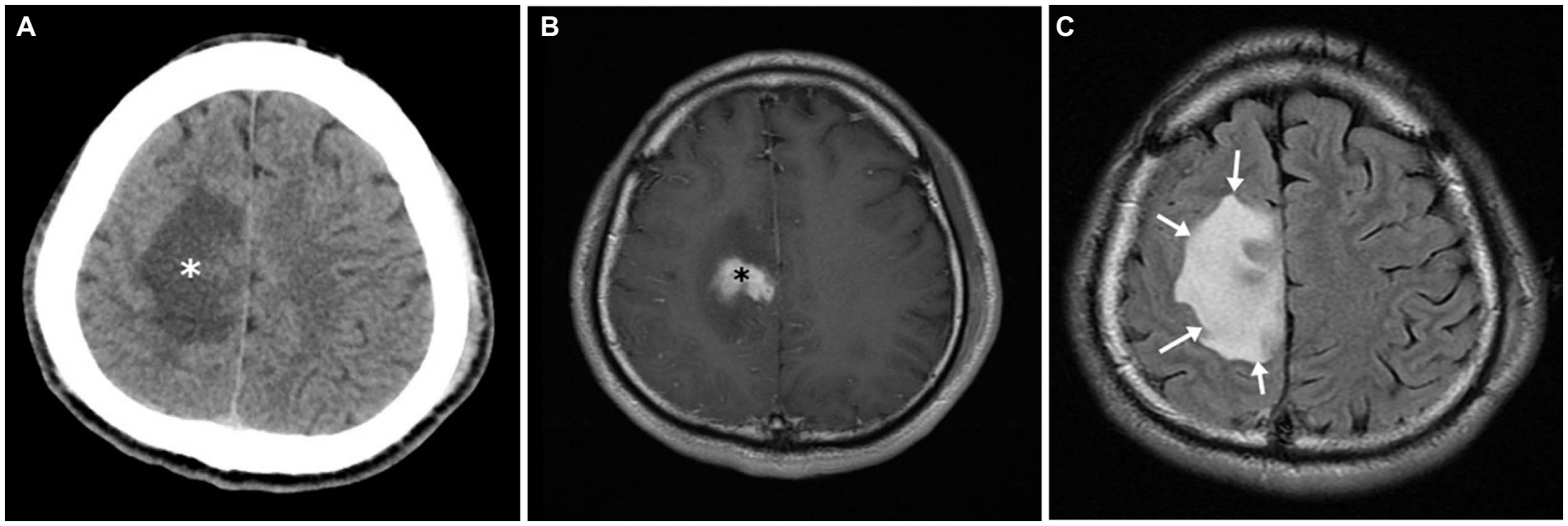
Central nervous system melioidosis of case 2. Unenhanced computed tomography (CT) showed a low attenuation in right frontal lobe (white asterisk) (A). Magnetic resonance (MR) images of the corresponding region depicted hyperintensity in the T2 weighted fluid attenuated inversion recovery (FLAIR) sequence (white arrows) (B) and an enhancing soft tissue lesion in the post contrast T1 weighted images (black asterisk) (C). All image findings are compatible with encephalitis.
